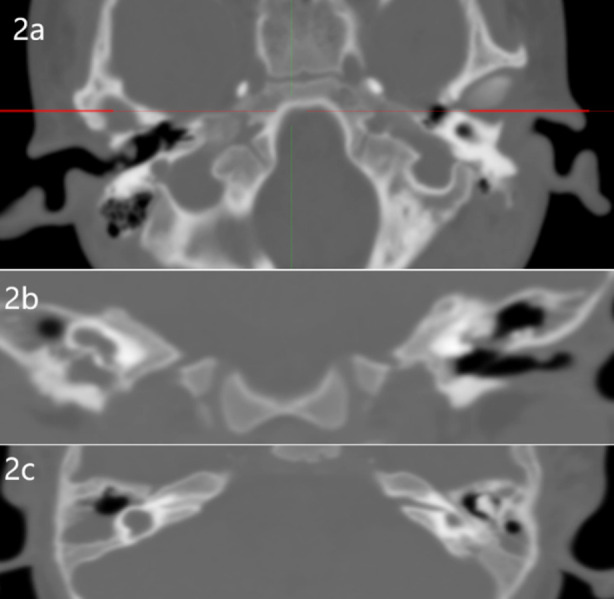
Computed Tomography Scan Axial view showing a) Congenital absence of inner ear & Vth Cranial Nerve bilaterally consistent with CLA, b & c) Hypoplasia of basal turns with 6mm on right side while 4 mm on left side with a cyst like structure replacing Semicircular canals consistent with CH 3.
